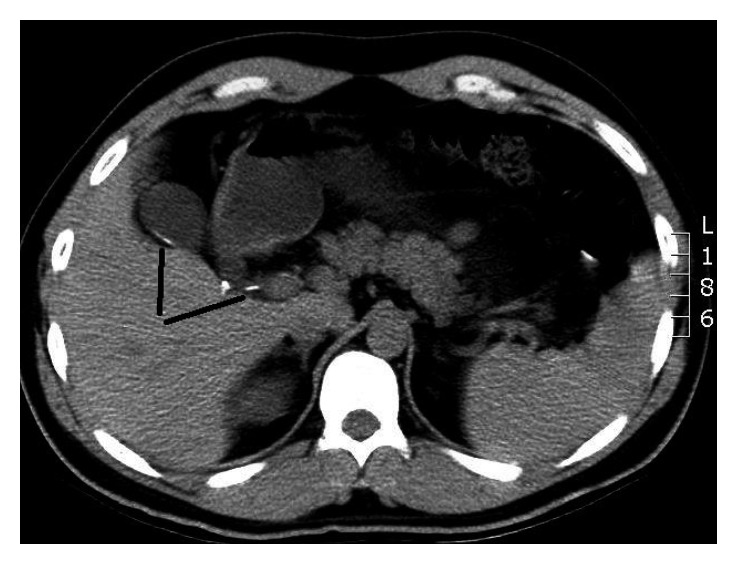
CT abdomen: linear calcification extending from the gallbladder through the cystic duct (dashes).
